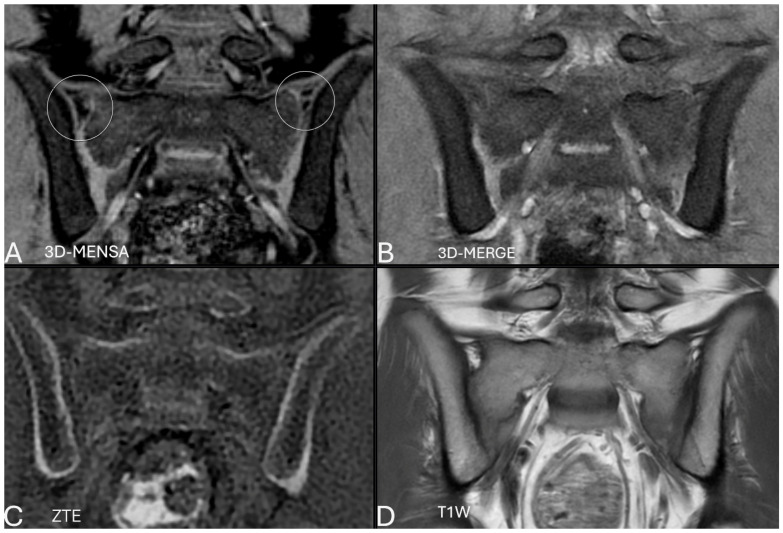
Coronal oblique MR images from a 12-year-old male patient were acquired from the same slice and time point using 3D-MENSA (A), 3D-MERGE (B), ZTE (C), and T1W (D) sequences. The 3D-MENSA (A) sequence allows for a clear distinction between the ligamentous component, the adjacent bone cortex, and the synovial component of the joint (white circles).
